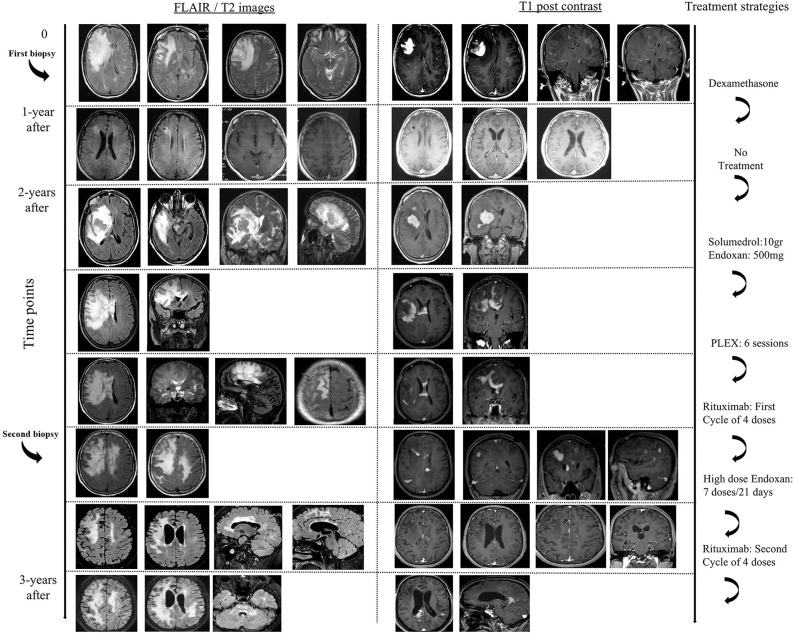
Brain MRI's in chronological order showing the effect of various treatment modalities.
